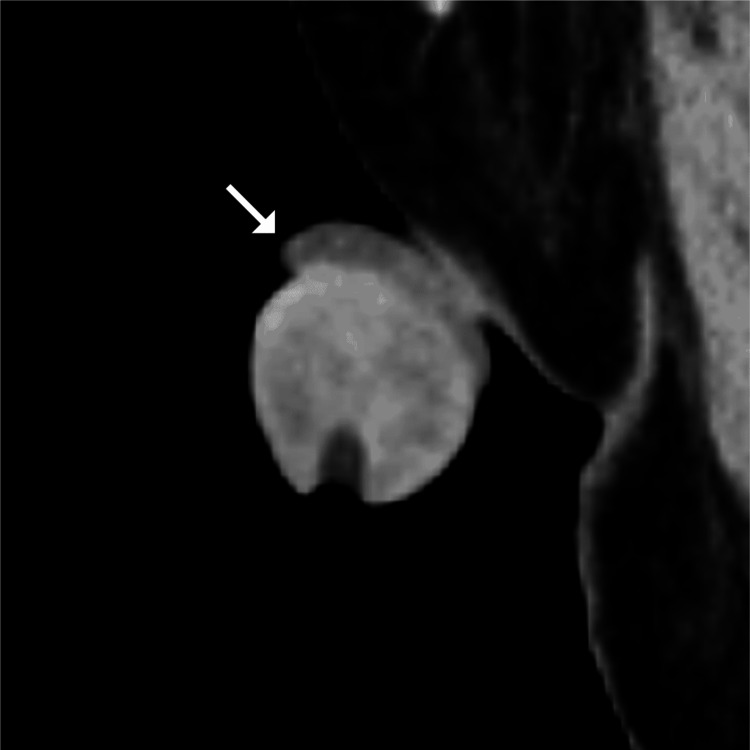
Sagittal contrast-enhanced CT of the penis. Sagittal contrast-enhanced CT of the penis shows a mildly thickened and hypoattenuating prepuce located proximal to the glans penis (long arrow).
